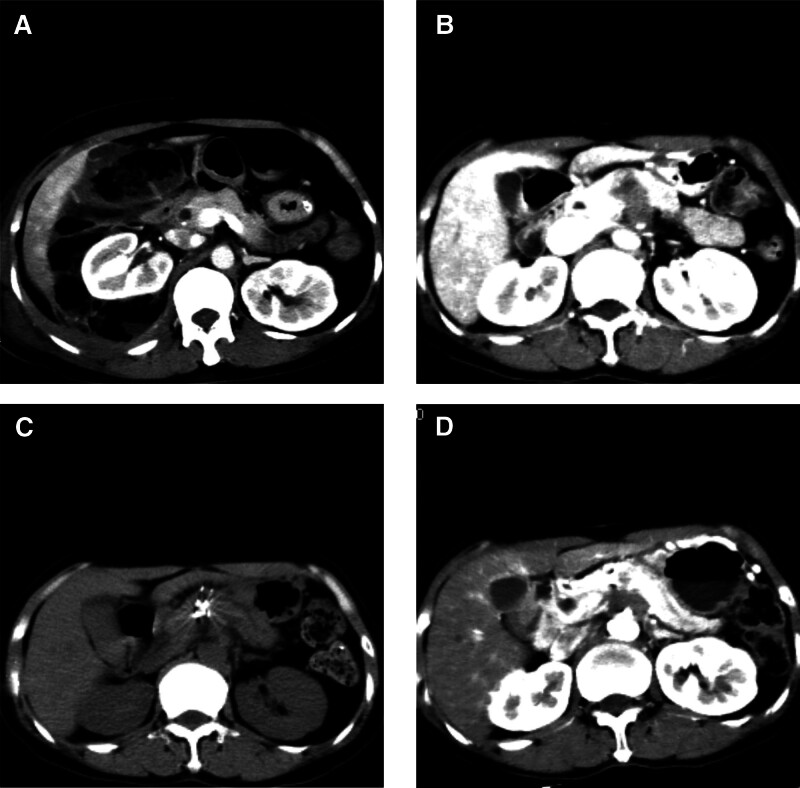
Abdominal computed tomography. (A) Normal image of the pancreas during initial colon cancer, (B) image of pancreatic metastasis when tumor relapses, (C) imaging of pancreatic metastatic cancer immediately after particle implantation, and (D) latest image of pancreatic metastases.
